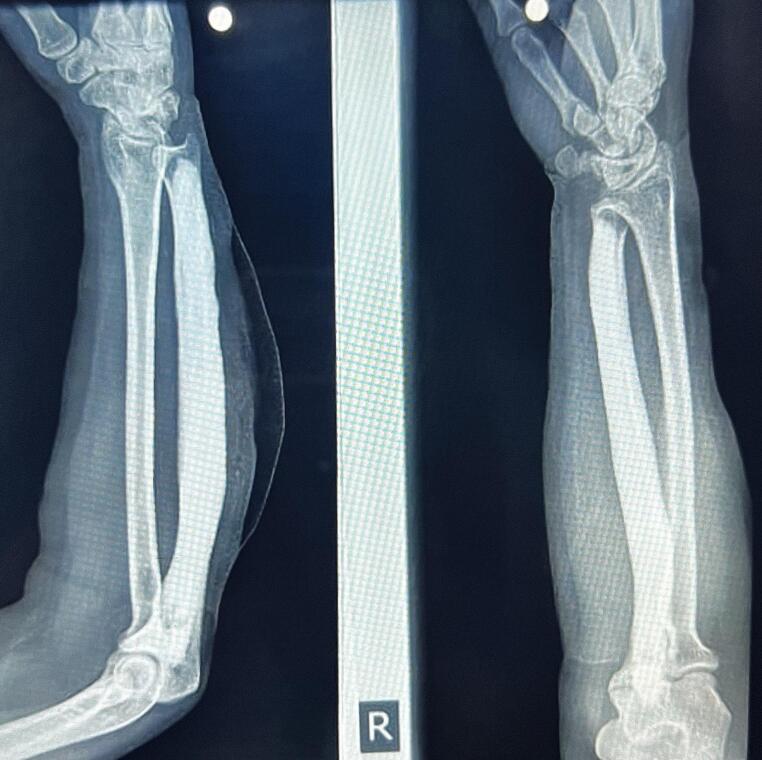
Postoperative radiograph of right forearm reshaped to normal anatomical contour of ulna after osteoplasty.
